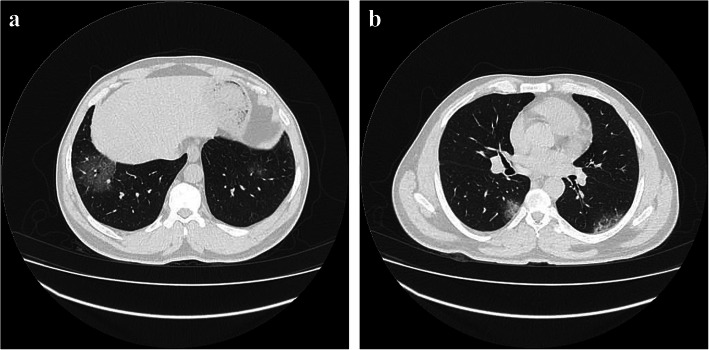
a Male, 14 years old. Chest CT showed bilateral basal ground glass opacities. b Male, 15 years old. Chest CT showed crazy paving pattern in both lungs
